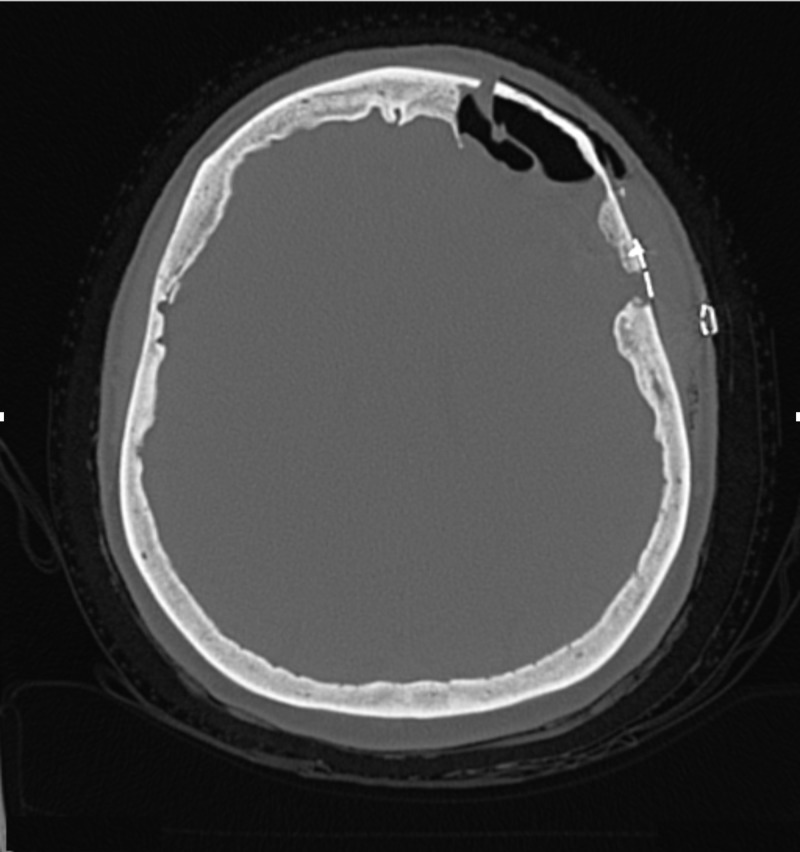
Postoperative computed tomography of the head
